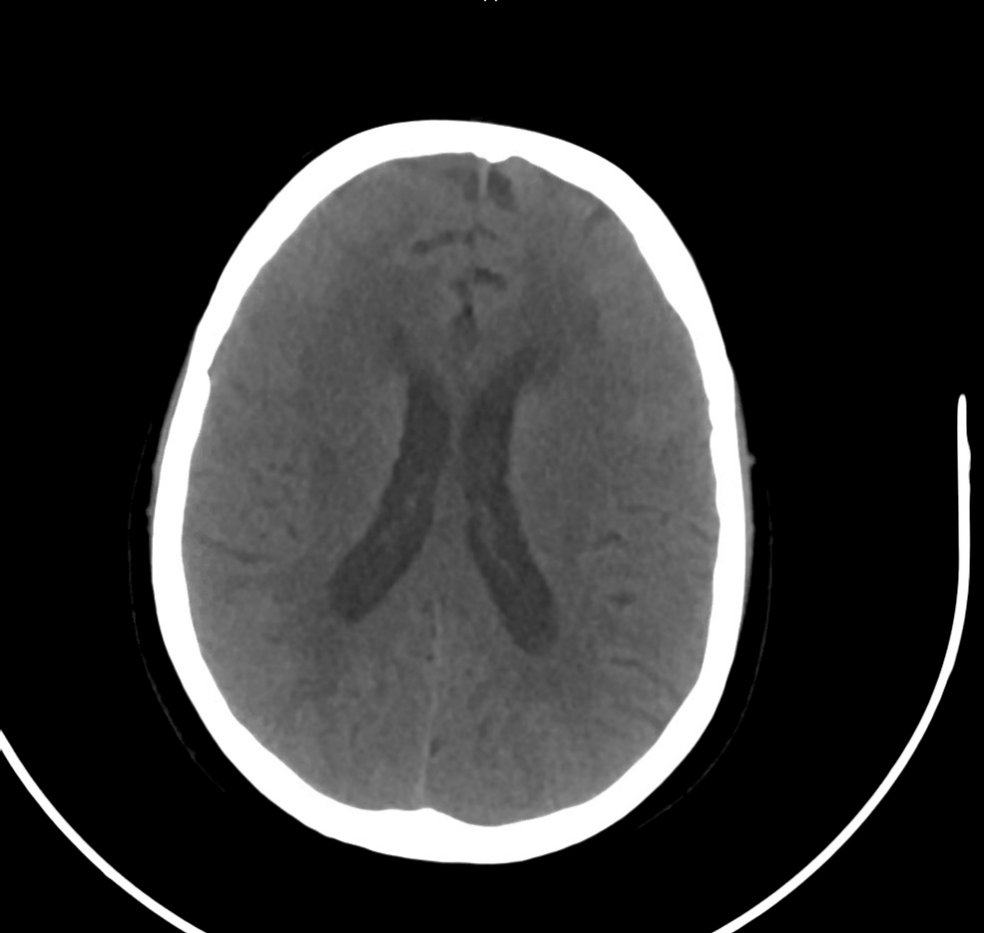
CT brain showing no definite mass lesions.
